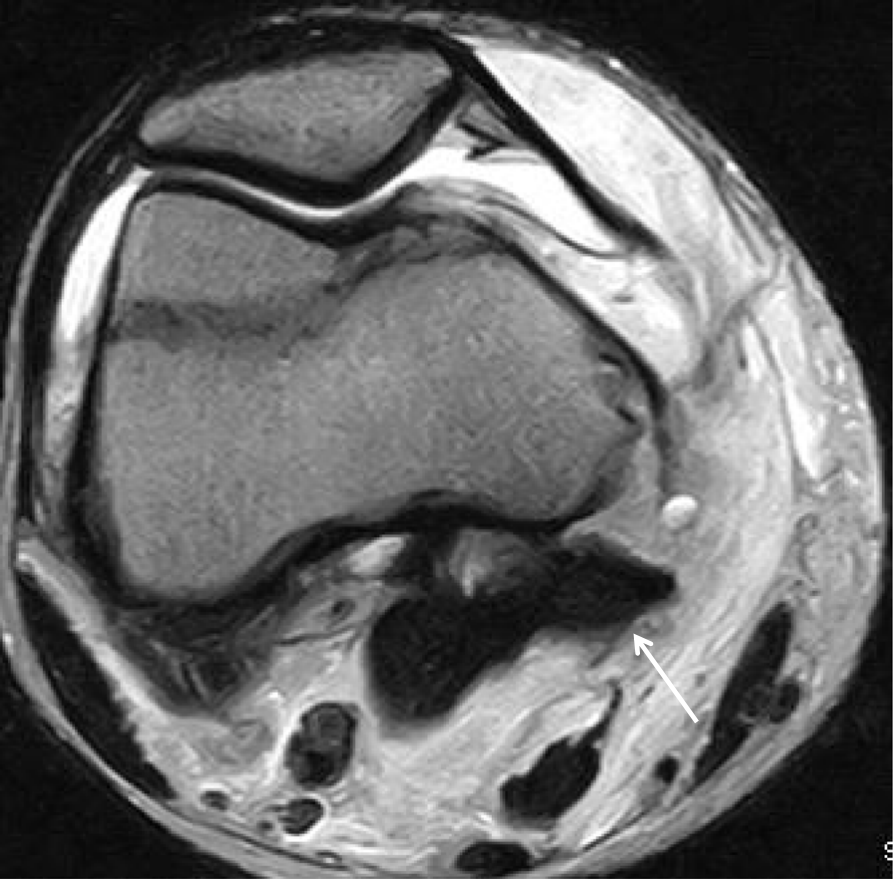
Magnetic resonance imaging of the injured knee. The axial view shows the bone fragment attached to the medial head of the gastrocnemius muscle (*arrow*)
